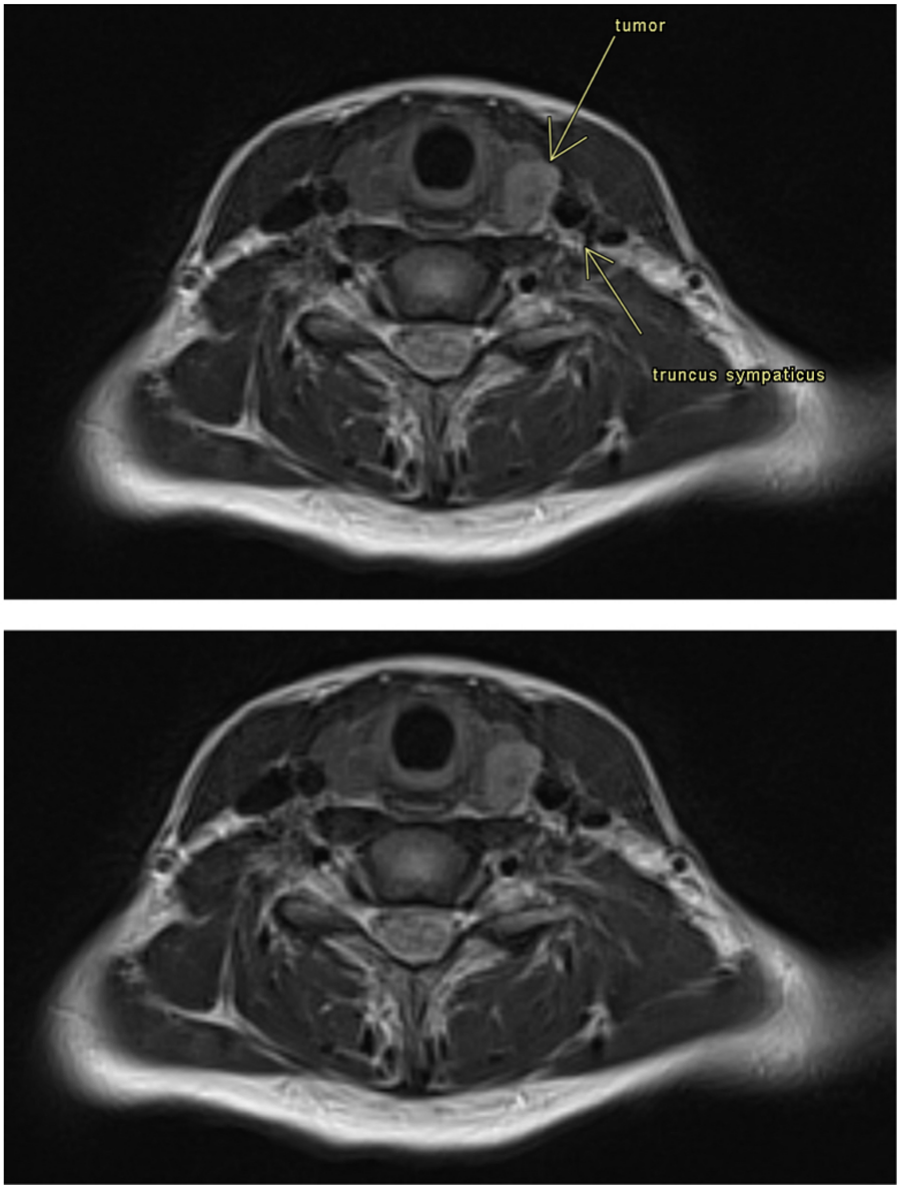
MRI was done on an Avanto Fit 1.5T (Siemens, Erlangen). The image is a transverse T2-weighted sequence with a thickness of 6 mm. The following were described by a neuroradiologist consultant: The image shows a tumor in the thyroid gland on the left side. There is no influence of the truncus sympathicus.
